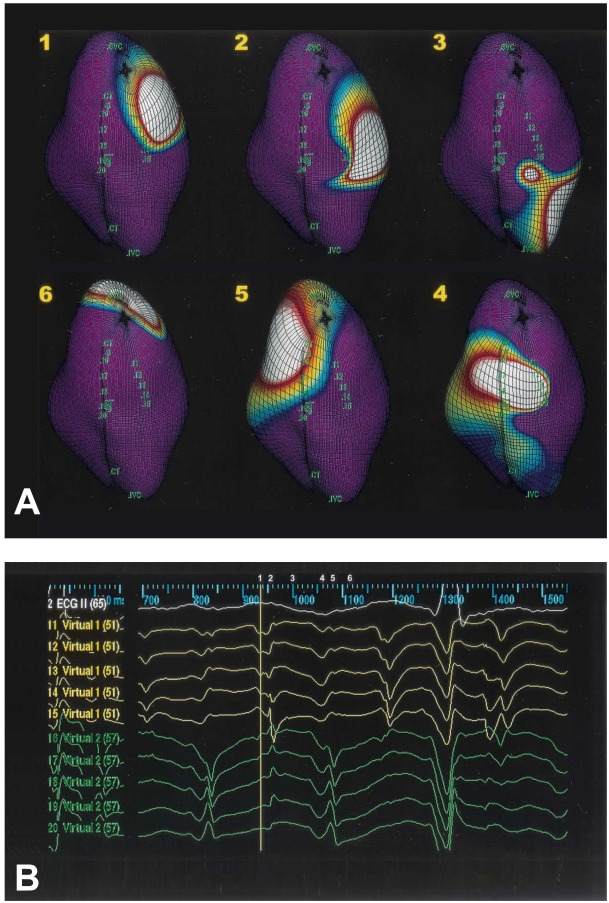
(A) Isopotential maps showing the activation sequence (frame 1 to 6) of counterclockwise upper loop reentry in the right posterior oblique view. Color scale for each isopotential map has been set so that white indicates most negative potential and blue indicates least negative potential. The activation wave front propagates down the anterolateral right atrium (RA) near the superior vena cava (SVC) (frame 1) to the middle and inferior anterolateral RA (frame 2), then splits into two wavefronts (frame 3); one passes around the area of functional block, and the other passes through the cavotricuspid isthmus. The wavefront in the lateral RA continue through the gap in the crista terminalis (CT) (frame 4) to the superior posterior RA (frame 5) and activates the atrial wall surrounding the SVC before reactivation of anterolateral RA once again. (B) The virtual electrograms from the area of functional block (virtual 11 to 15) and the CT (virtual 16 to 20) including the conduction gap (virtual 16 to 18) demonstrate double potentials. The numbers 1 to 6 represent the time points at which the isopotential maps have been displayed in A. IVC = inferior vena cava. (Reproduced from Tai CT, Huang JL, Lin YK, et al. Noncontact three-dimensional mapping and ablation of upper loop reentry originating in the right atrium. J Am Coll Cardiol 2002;40:746-753, with permission from the American College of Cardiology Foundation.)
